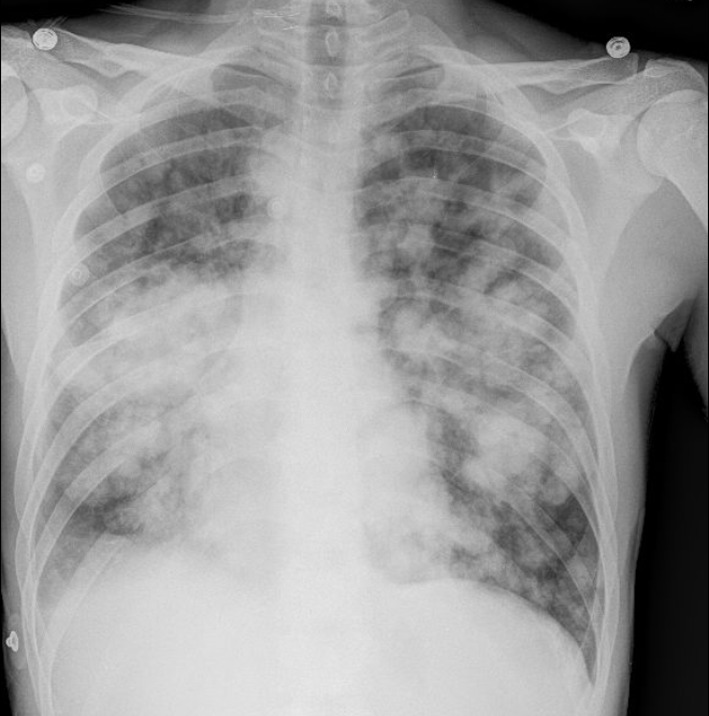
Chest X-ray showing bilateral rounded airspace opacities.
